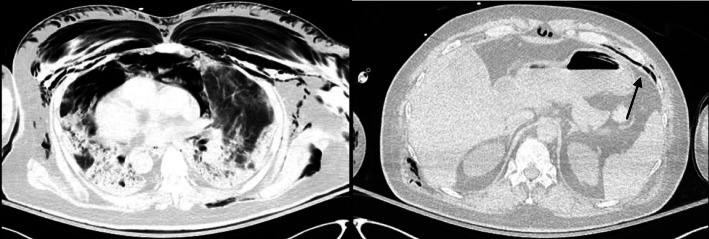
Computed tomography of the chest without contrast of Patient 2. A large pneumomediastinum and extensive soft tissue emphysema involving the anterior and posterior thorax (left). A tiny pneumoperitoneum in upper anterior abdomen (right)
